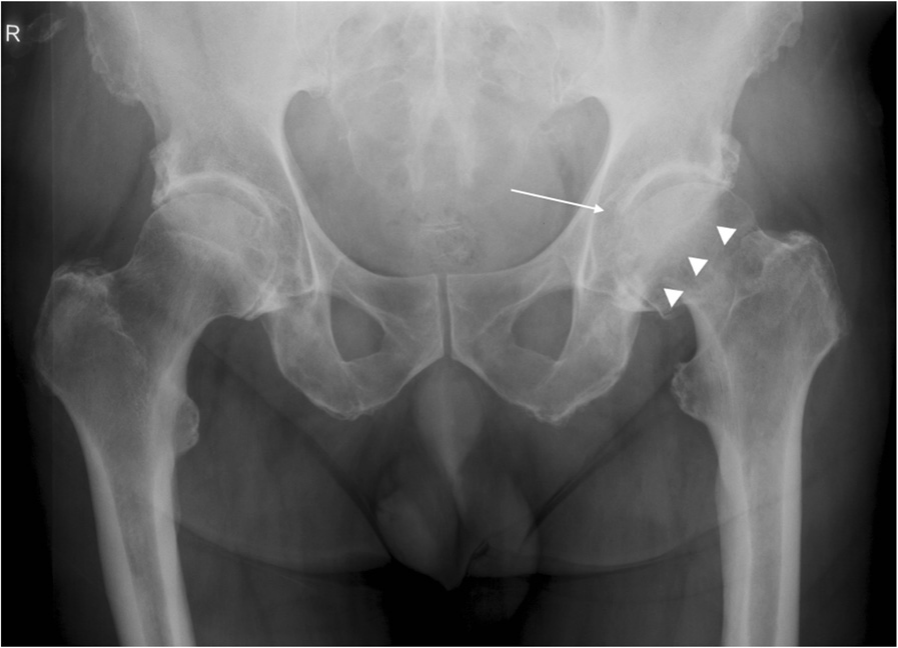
Pelvic X-ray with signs of arthrosis consisting of a double fond osteophyte (DFO) (*thin arrow on the left*) and a posterior wall sign (PWS) (*thick arrows on the right*)
